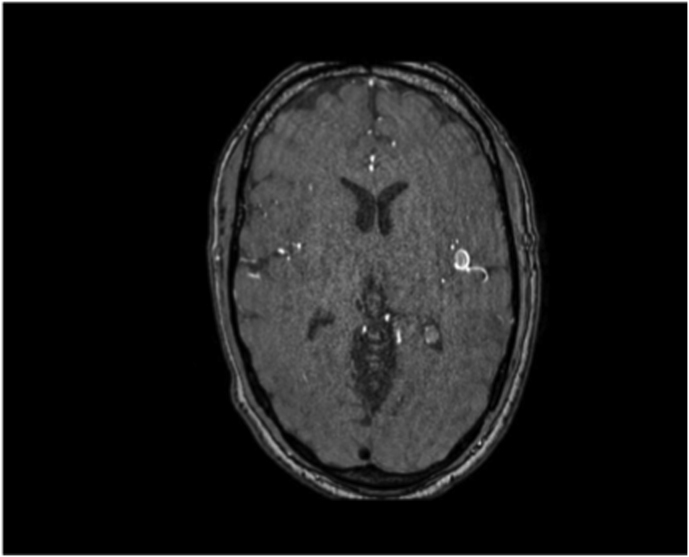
Diagnostic Images of Case #3*. A Diagnostic DSA, ICA injection, lateral view showing the distal fusiform MCA aneurysm. B) Diagnostic MRI depicting the deep location of the aneurysm and the efferent branches within the distal Sylvian fissure.
